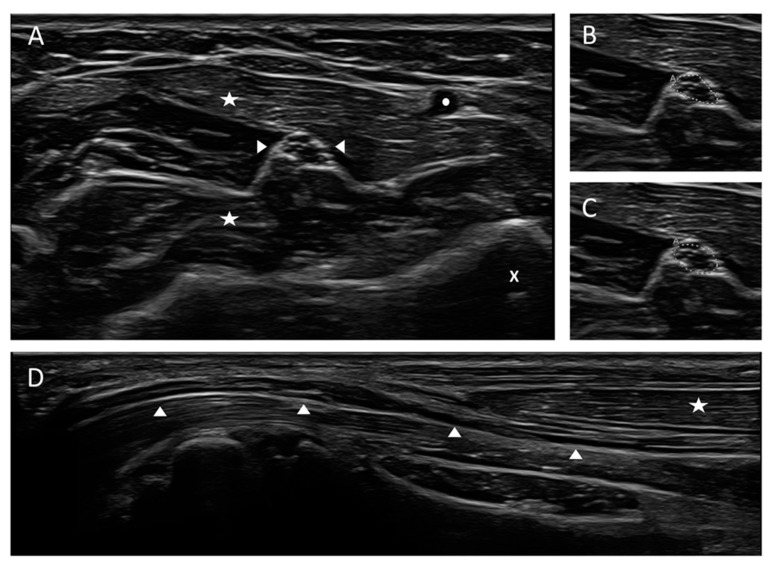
Cross-sectional and longitudinal view of a normal median nerve at forearm level. (A) Cross-sectional view of the median nerve. The nerve appearance is honey-comb-like with hypoechoic fascicles and hyperechoic epi- and perineurium. (B,C) Measurement techniques of the cross-sectional area (CSA) using the free-hand (B, upper picture) or ellipse function (C, lower picture) of the ultrasound device, CSA 5 mm^2^. (D) Longitudinal view of the median nerve in panoramic view. Left side of the picture: entrance into carpal tunnel. Arrow heads are marking the nerve, asterisks the superficial and flexor digitorum muscles, the circle the radial artery and the x the radial bone, “A” in pictures B and C means “area”.
